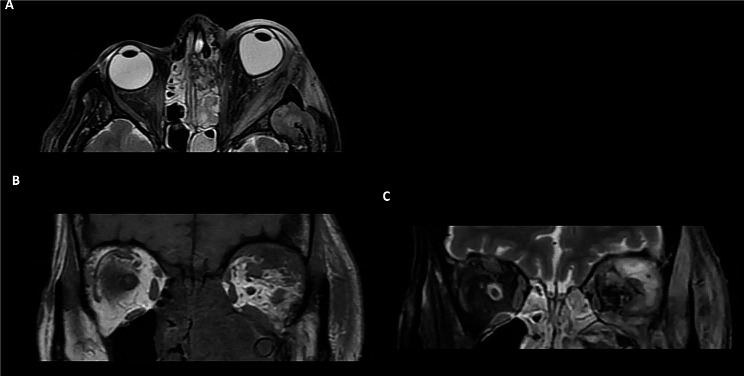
Case 4: A: MRI image demonstrating left sided proptosis with tenting of the globe. B-C: There is an abscess centred around the left superolateral and inferior orbit that is T1 isointense and T2 hyperintense, with associated enlargement and oedema of the lateral and inferior recti
